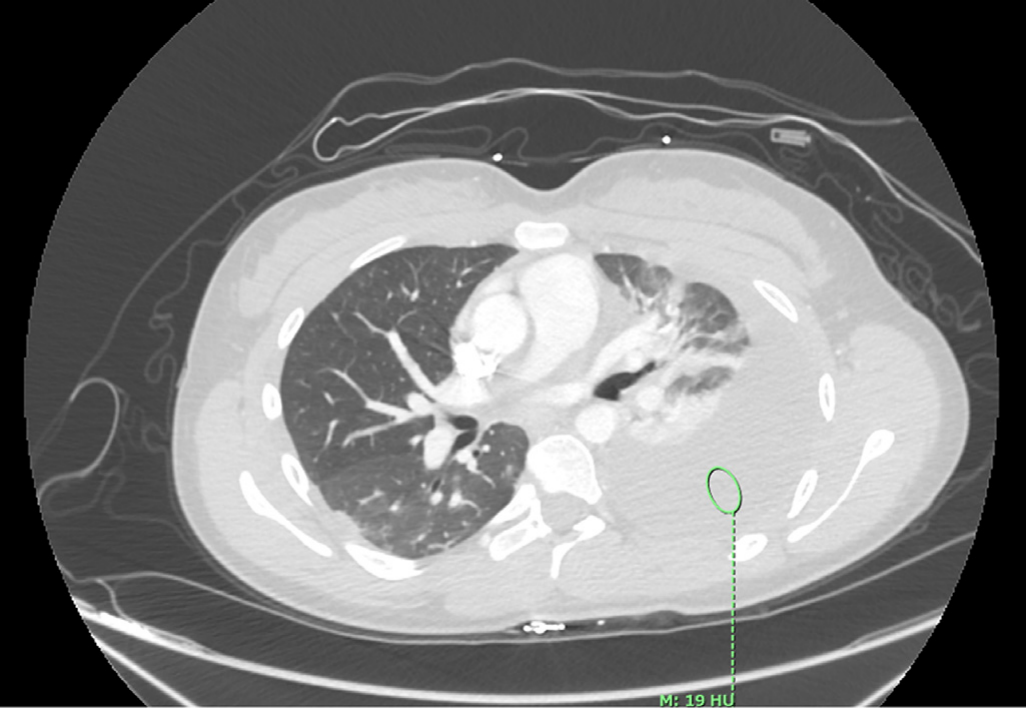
CT chest shows low density large pleural effusion (circle).
